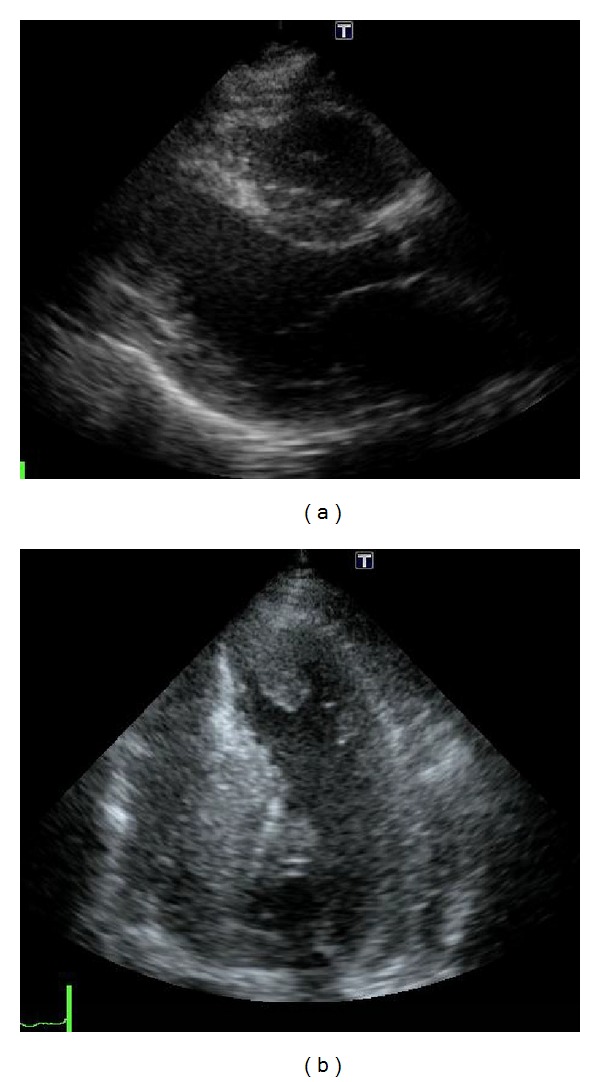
Parasternal long-axial view (a) and four-chamber view (b) of the transthoracic echocardiography showing asymmetrical hypertrophy and a thin-walled apical aneurysm with thrombus.
